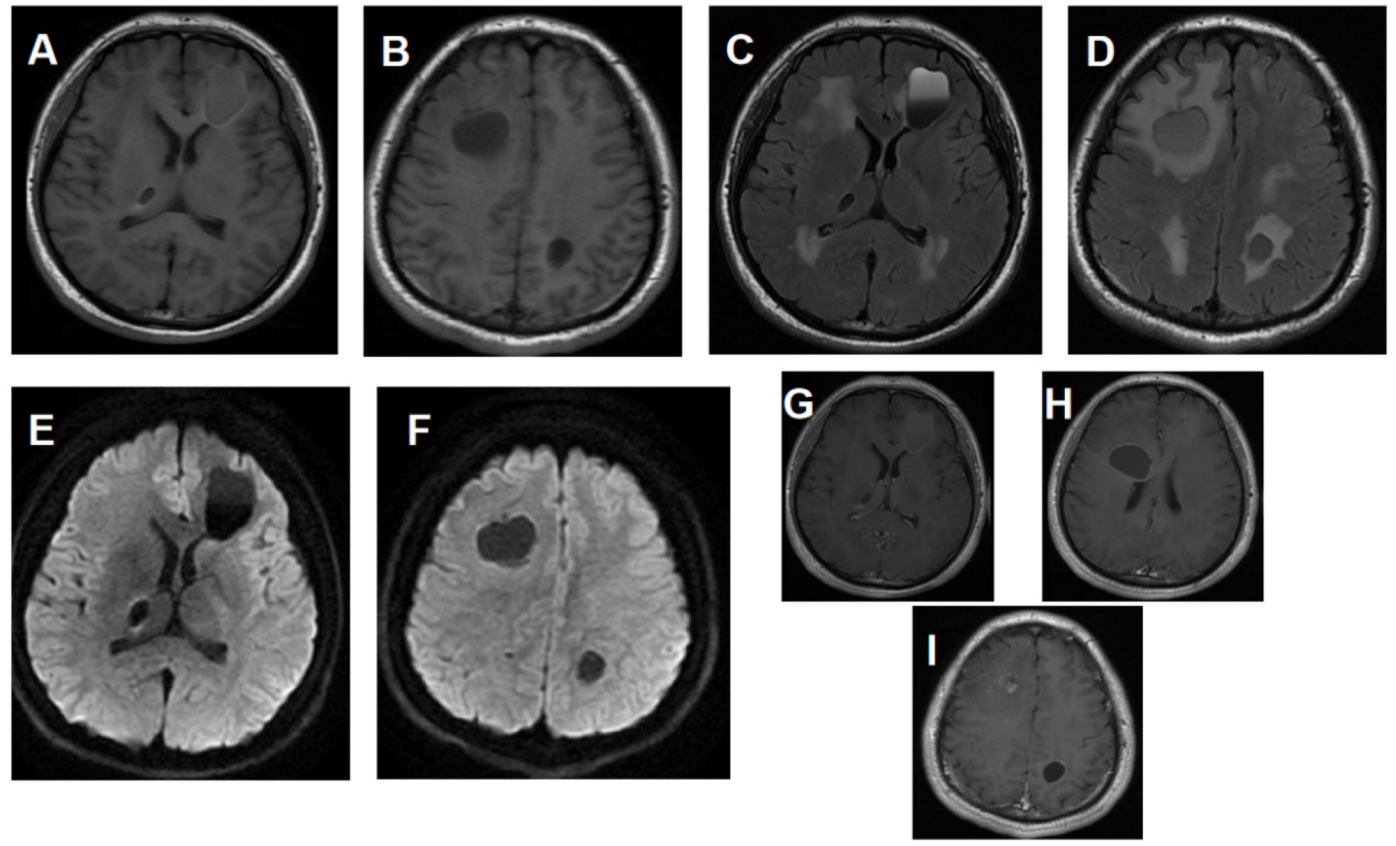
Cranial MRI and enhancement at the time of patient’s visit in April 2019. (A) MRI T1 shows fluid signals in the right frontal lobe and left parietal lobe, accompanied by edema of surrounding brain tissue. (B) MRI T1 reveals mixed signal shadows in the left frontal lobe and low-density shadows in the right thalamus. (C,D) MRI T2 shows cysts in the right frontal lobe and left parietal lobe, nodular low signal shadows in the right thalamus, and solid cysts in the left frontal lobe. (E,F) MRI diffusion-weighted imaging shows cystic lesions with restricted diffusion. (G–I) MRI enhancement shows multiple white matter lesions, with no enhancement observed in the cysts.
